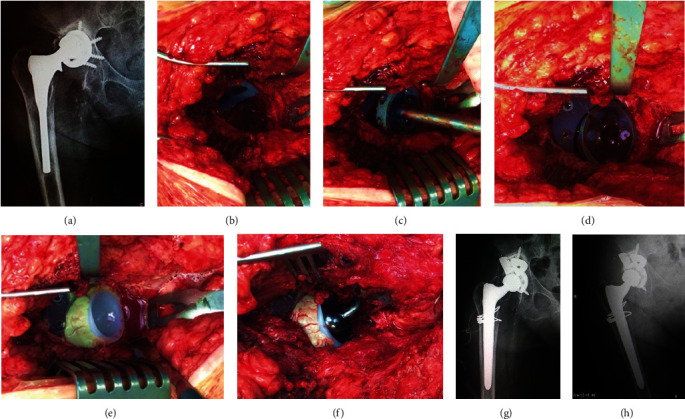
(a) Preoperative X-ray. (b) “Footing” type augment at the cavitary defect. (c) Cup insertion. (d) Cup-augment construct. (e) Polyethylene insertion. (f) Intraoperative image after reduction of the hip. (g) Postoperative X-ray. (h) 5 y follow-up.
